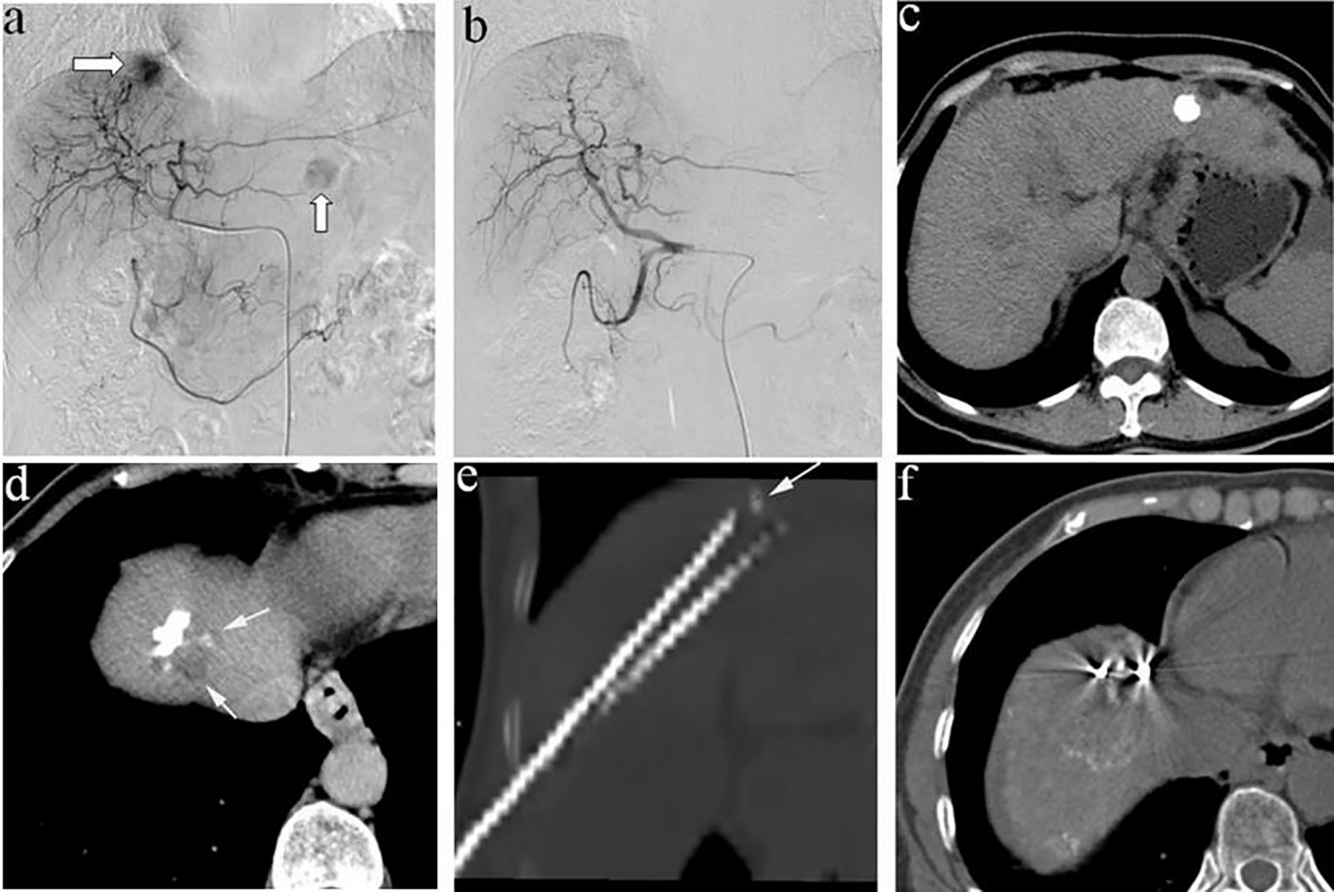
(A) A 58-year-old man, angiography revealed two tumors in the liver, one located at the diaphragm dome and the other at the left lobe; (B) Complete embolism achieved with TACE; (C) CT examination 2 weeks after TACE showed that the lesion located at the left lobe had good iodide deposition, (D) while the tumors located at the diaphragm dome had poor iodide deposition and small residual lesions; (E) therefore, the patient underwent ^125^I seeds implantation, and (F) plain CT scan after ^125^I seeds implantation showed good seeds distribution.
